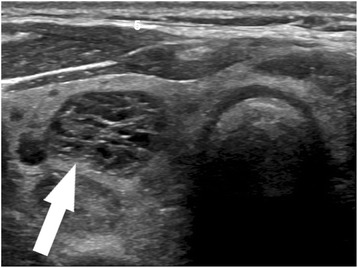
Very low suspicion pattern. Transverse grayscale sonographic image of the thyroid at level of thyroid isthmus shows a nodule in the right lobe (*arrow*) with a “spongiform” pattern. Note the innumerable tiny cystic spaces characteristic of this pattern
